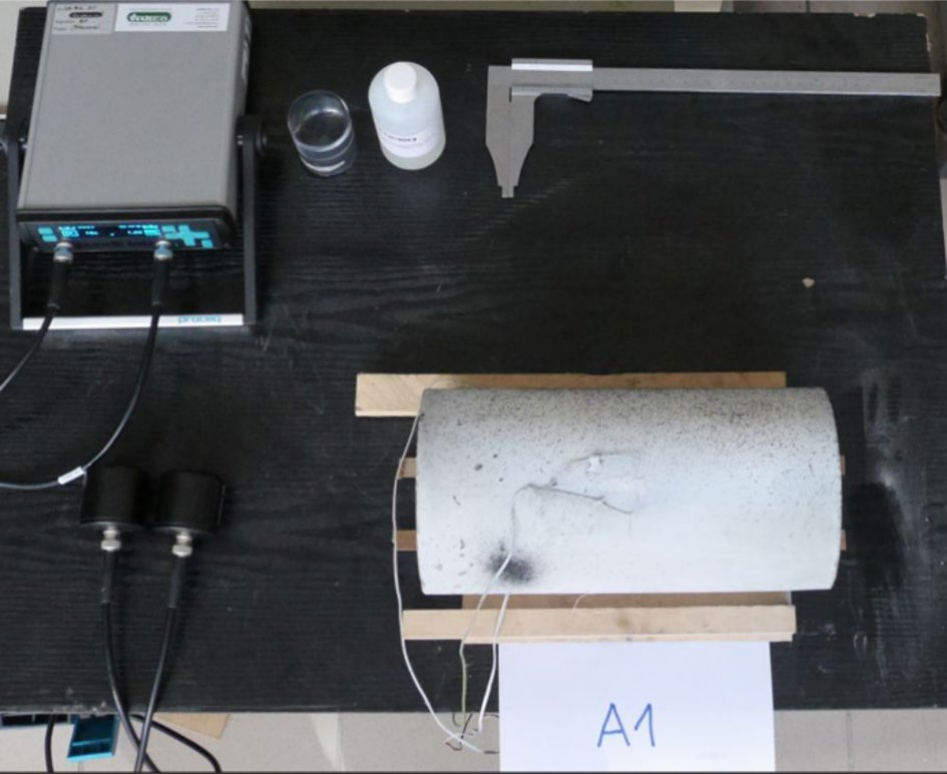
Experimental setup for testing samples by ultrasonic method.
